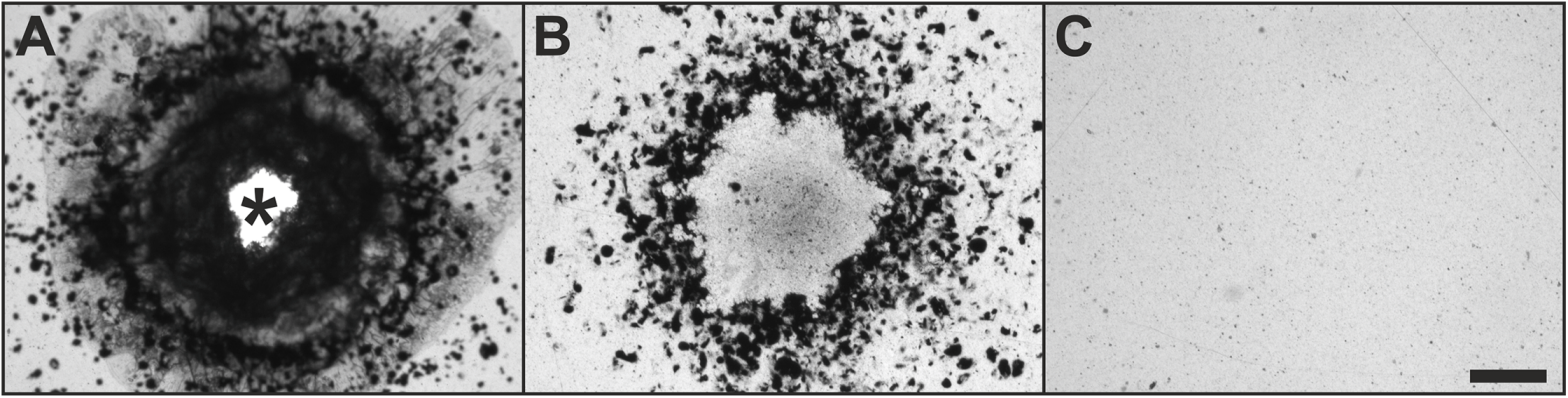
Damage of the surface of x-ray film caused by pressure waves generated by the D-Actor 200 and the Swiss DolorClast devices. The figures show the surface of x-ray film after exposure to 10,000 pressure waves generated by the D-Actor 200 (A) and the Swiss DolorClast (B) at maximum energy settings (i.e., 5 bar for the D-Actor 200 and 4 bar for the Swiss DolorClast). The asterisk in (A) indicates a hole in the x-ray film. The Vibracare device operated at maximum energy settings (50 cycles per second) had no detectable impact on x-ray film (C). The scale bars represent 500 μm.
